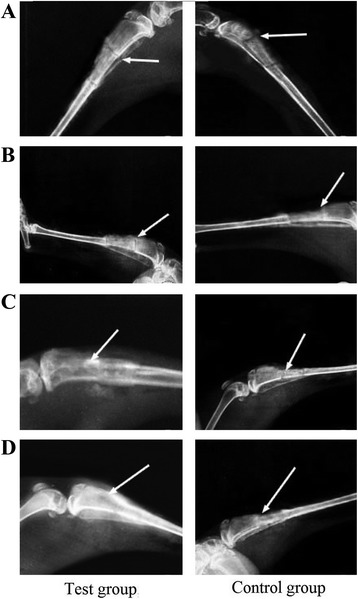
X-ray examination after surgery. a 4 weeks after surgery. b 8 weeks after surgery. c 12 weeks after surgery. d 16 weeks after surgery
